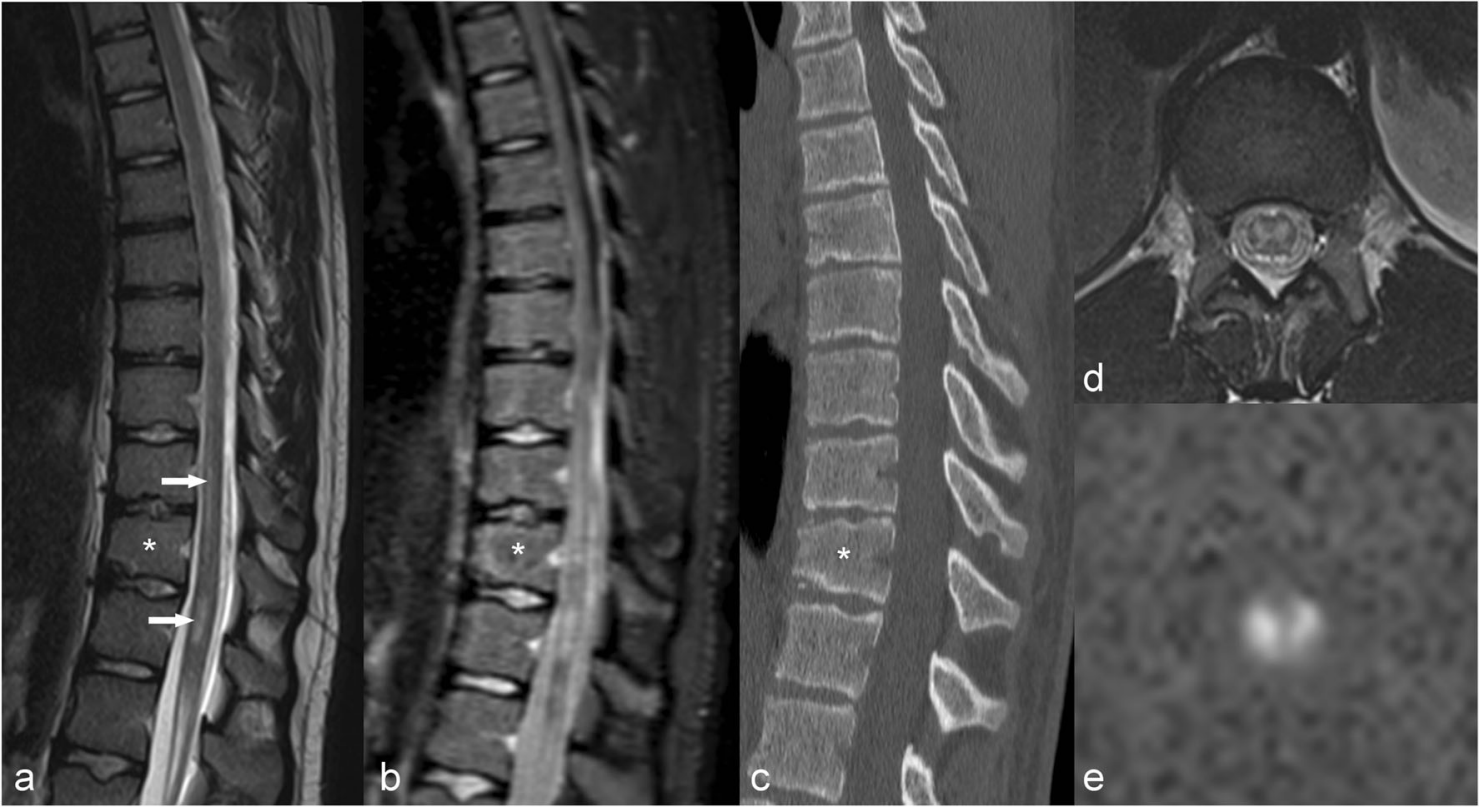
A 14-year-old male who suffered minor trauma. Spine MR performed 24 h later shows increased signal intensity on T2WI (a,d) (arrows) and DWI (e) reflecting ischaemia. Note an acute wedge fracture of T12 (*)
